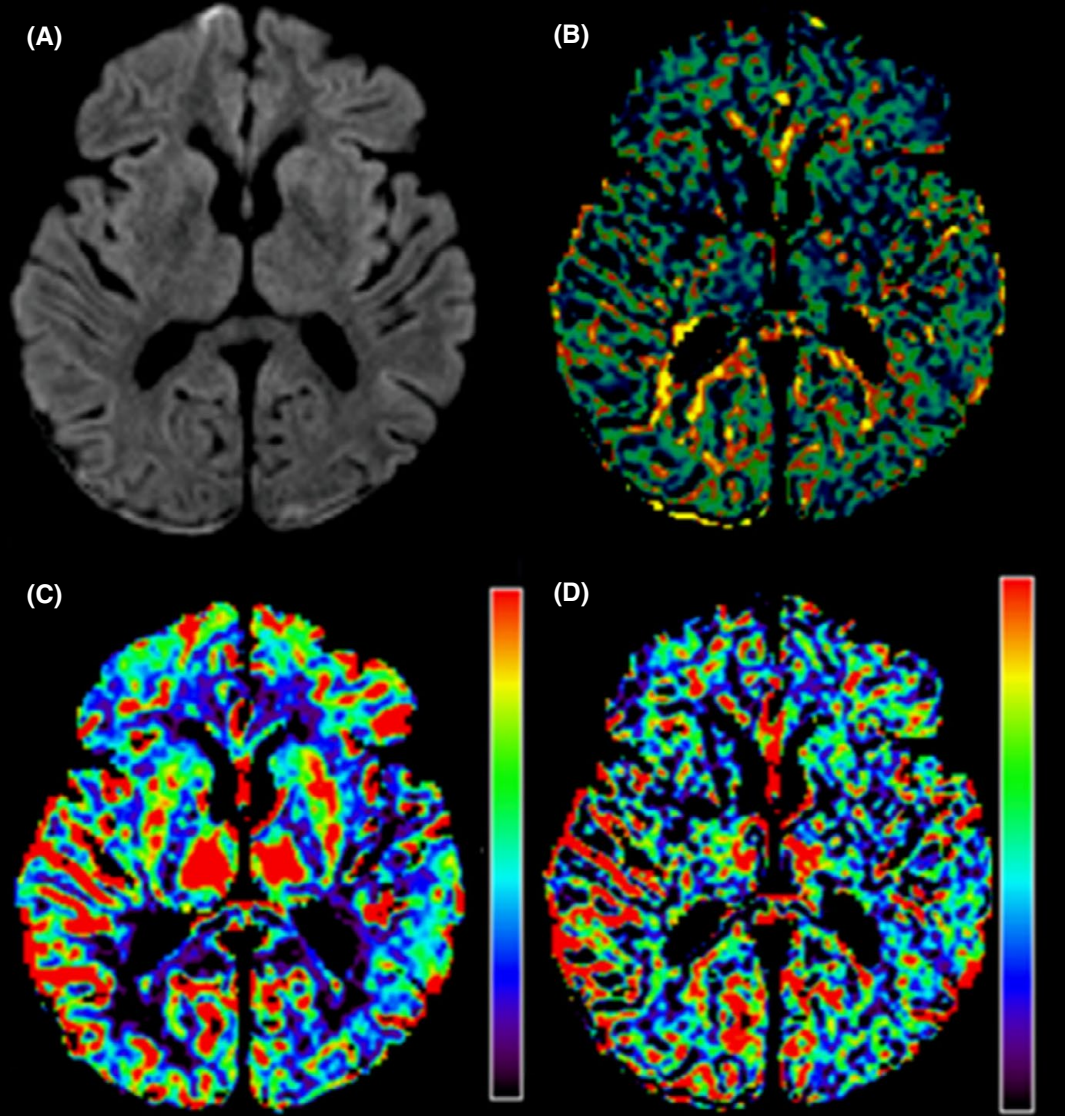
Follow‐up magnetic resonance imaging 5 hours after the initial computed tomography exam showing no diffusion restriction in the diffusion‐weighted imaging sequence (A). Note the bilateral occipital subdural hematoma. The MR perfusion shows normalization of the perfusion parameters with reversible changes in mean transit time (MTT) (B) and cerebral blood flow maps (C). No changes are apparent in the cerebral blood volume map (D)
